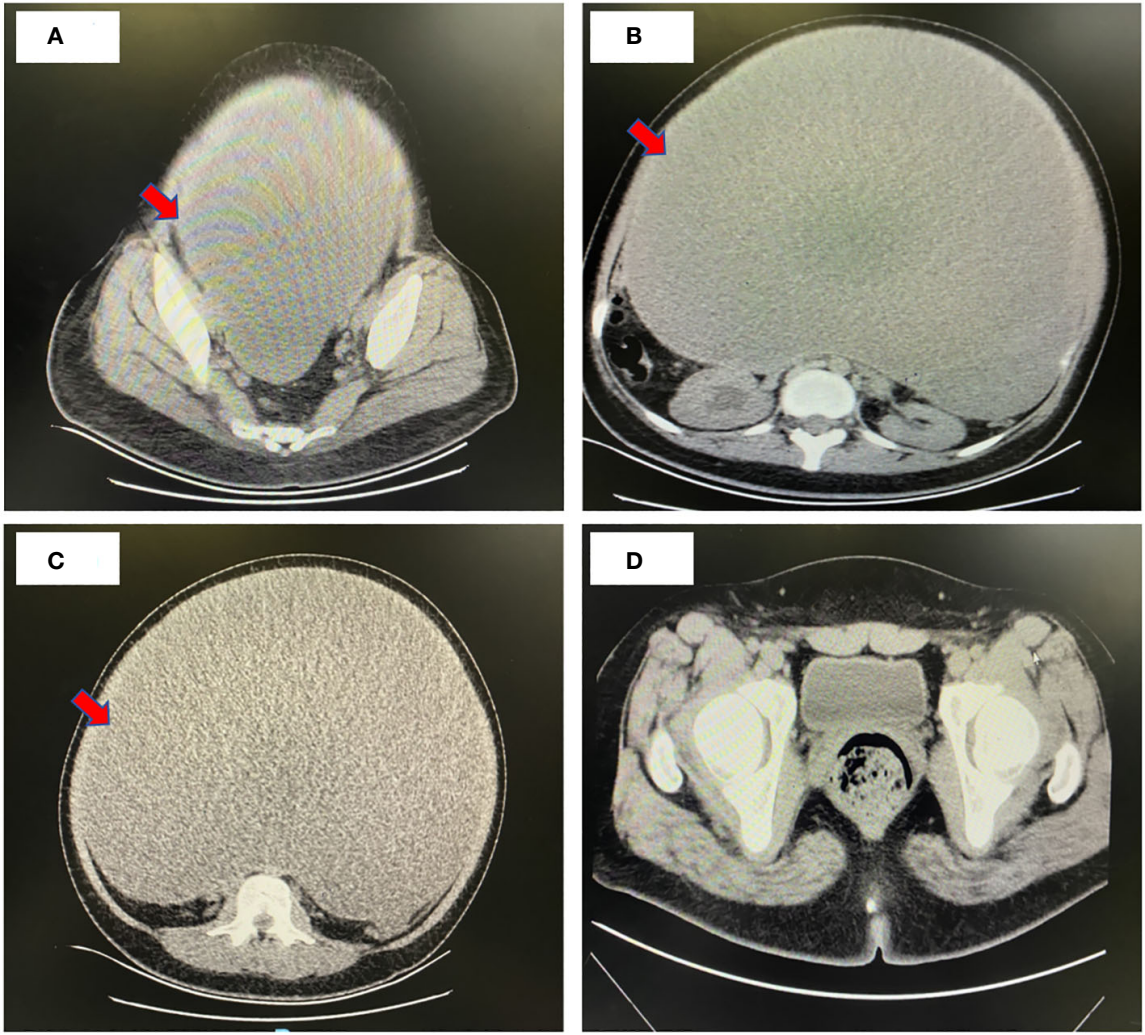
Whole-abdomen enhanced computed tomography image. (A-C) Preoperative CT findings: Abdominal distension and a substantial cystic fluid lesion in the abdomen measuring approximately 40 cm x 30 cm x 34 cm. Contrast enhancement revealed mild strengthening of both septum and capsule wall, with no internal fluid density shadow enhancement at any stage. No distinct limited thickening of the septum was observed. Uterine compression and leftward displacement, along with compression of the abdominal aorta and adjacent intestinal lumen, were noted. Right renal corpuscle and calyces exhibited dilatation and hydronephrosis, as did the upper part of the right ureter. Provisional diagnosis: Massive cystic presence in the abdominopelvic cavity, suggestive of a cystic tumor likely of right adnexal origin, possibly a plasma cystadenoma. Right upper ureter compression was implicated in right lower ureter dilatation and hydronephrosis. (D) Postoperative CT findings: Changes in the right ovary, with a few striated shadows and effusion in the operative area. Discernible uterine morphology, and the left adnexa appeared replete.
